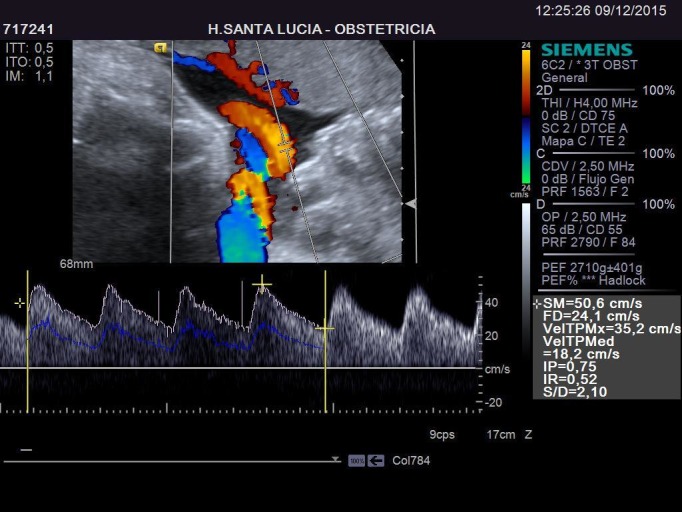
Umbilical Artery Doppler in Week 35
